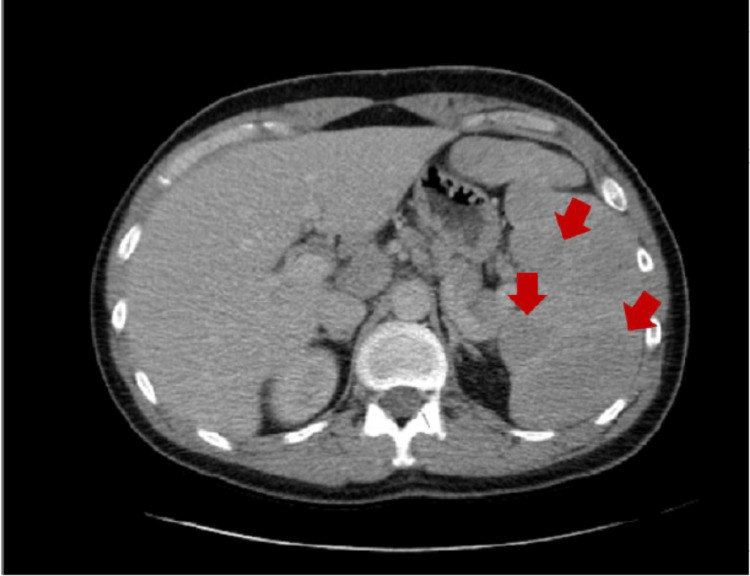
CT of the abdomen with contrast. This is a transverse CT cross-section showing an enlarged spleen with multiple hypoattenuating lesions (red arrows).
